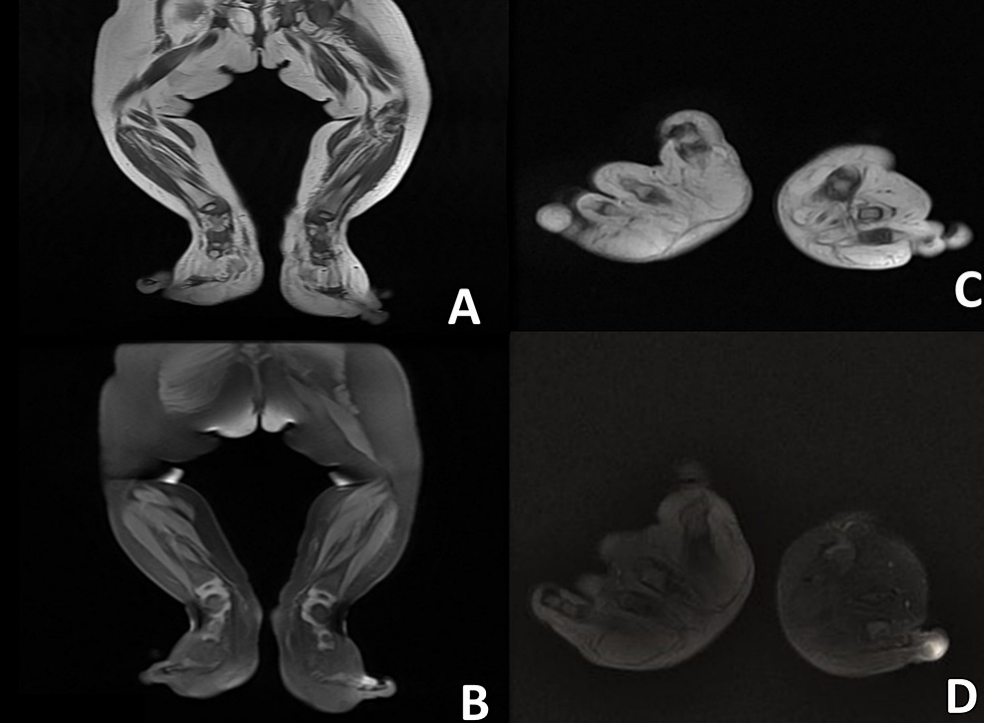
MRI image. Coronal T1W (A), coronal fat-suppressed sequence (B), axial T1W (C) and axial T1 fat suppressed (D) showing accumulation of excessive fat in the subcutaneous tissue without discernible capsule. Fibrous strands are seen within the fat in bilateral feet both in plantar and dorsal aspect (more in plantar aspect). There is osseous hypertrophy and bony overgrowth without any cortical thickening
